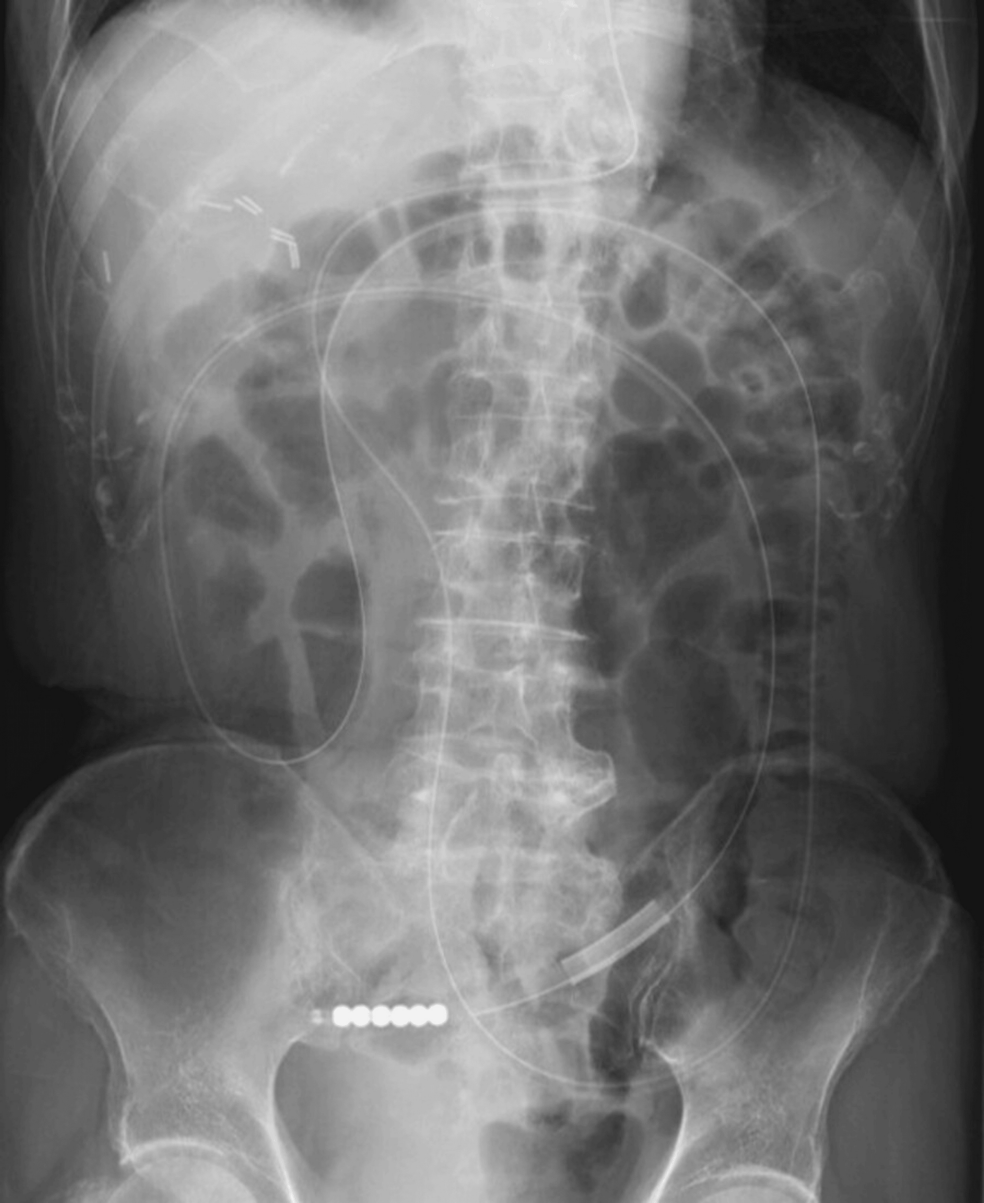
A follow-up abdominal radiograph confirmed resolution of the volvulus Follow-up abdominal radiograph on hospital day 7 showing resolution of the cecal volvulus. The previously dilated cecum has returned to normal caliber, confirming successful decompression after transnasal ileus tube placement.
